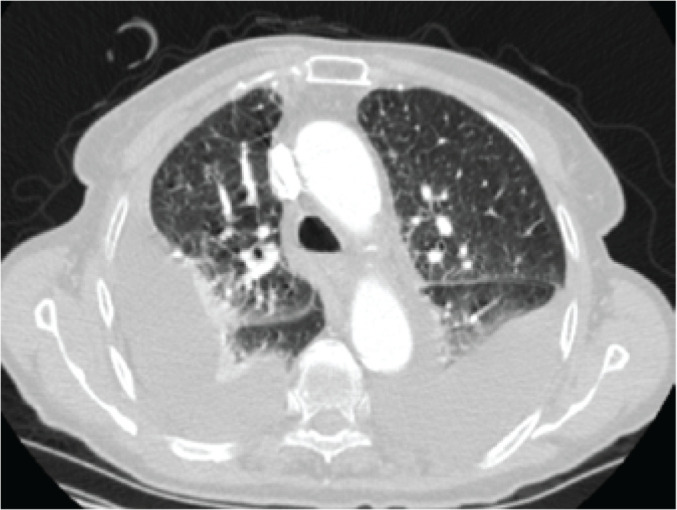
Full body computed tomography.
